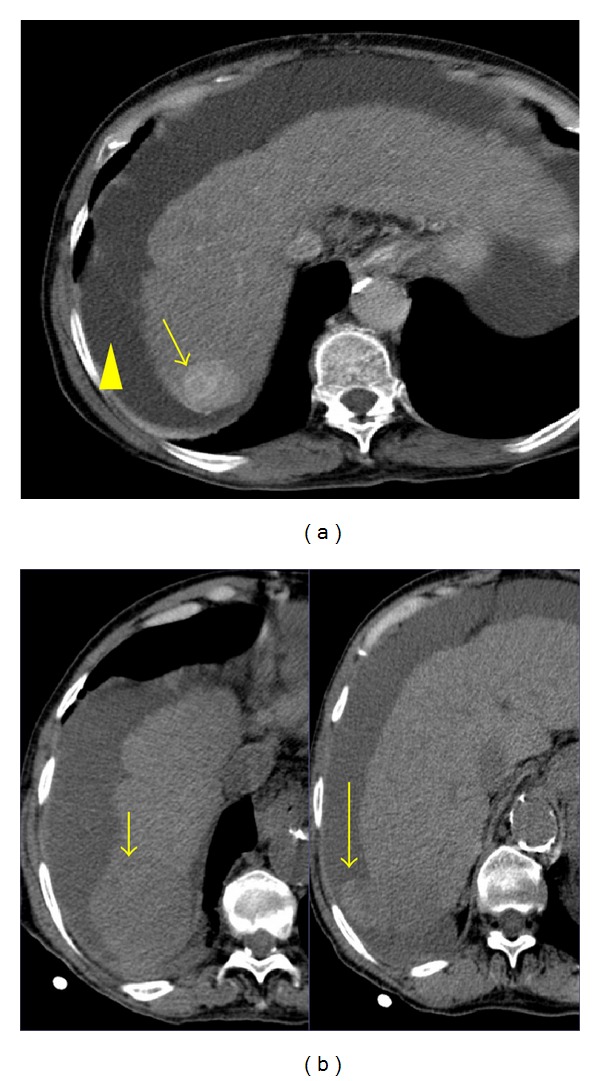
Ruptured HCC. (a) Contrast-enhanced CT shows a focus of HCC in the right lobe of the liver (long arrow). Low density fluid in the perihepatic space is also seen (arrowhead). (b) Three months later, the patient complained of increased abdominal pain. A nonenhanced CT obtained at the same level as (a) demonstrates an abnormally shaped right hepatic lobe (short arrow) associated with high density ascitic fluid consistent with hemoperitoneum (long arrow). Ruptured HCC was confirmed at surgery.
